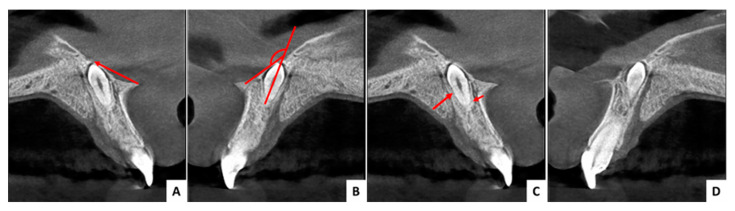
CBCT analysis: (A) distance from the ANS highlighted by the red arrow, (B) angle formed by the mesiodens axis with the nasal floor highlighted by the red lines, (C) distance to the mesiodens from the buccal and palatal aspects highlighted by red arrows, (D) position regarding the nasopalatine canal.
